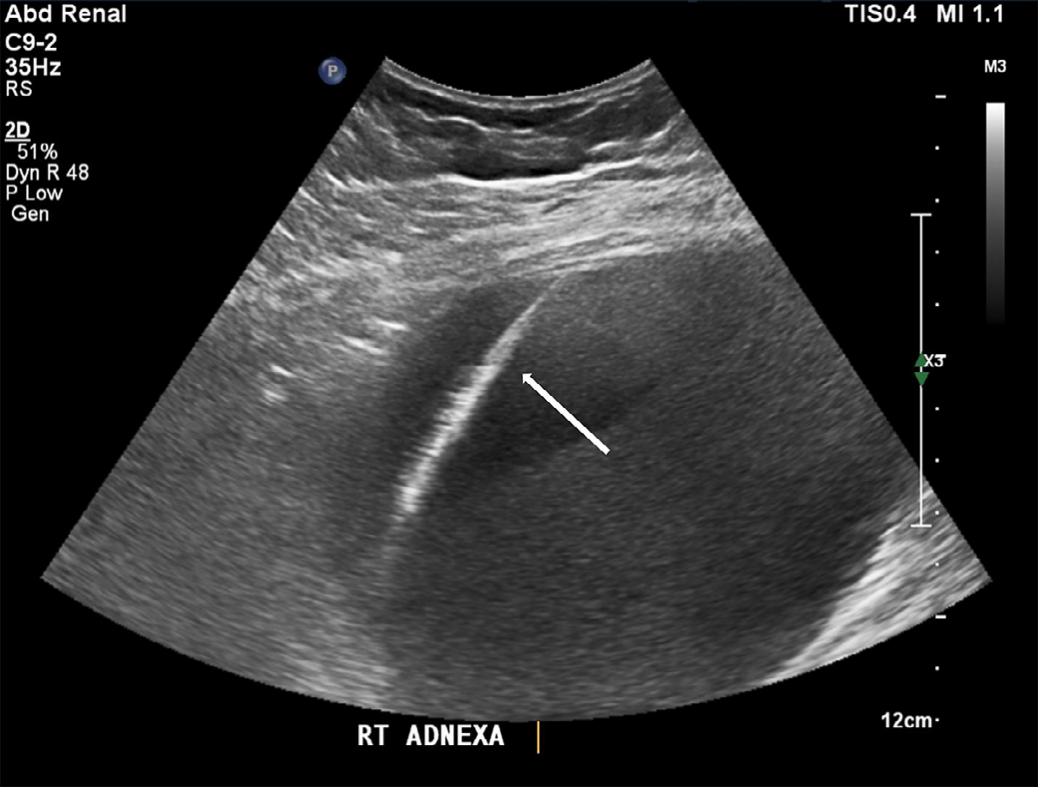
Transabdominal ultrasound of the pelvis with percutaneous catheter placement of the right adnexa demonstrates a fluid fluid with wire placement (arrow).
